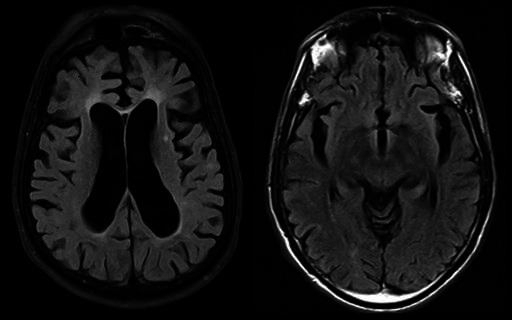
Non-contrast MRI brain showing atrophic changes in bilateral frontotemporal lobes.
